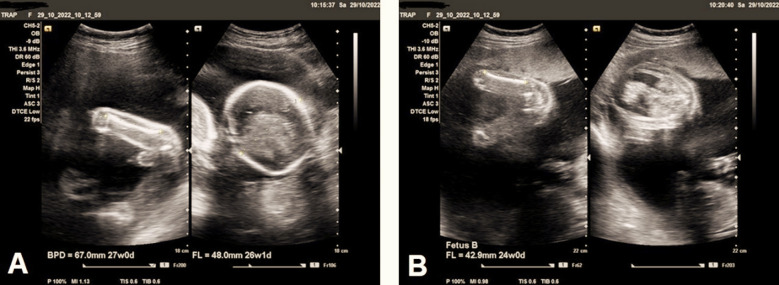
(A) Ultrasound imaging study shows the gestational age of the pump twin as 27 weeks by biparietal diameter (BPD), and 26 weeks+1 day by femur length (FL), and 27 weeks by abdominal circumference (AC), with about 983 gram expected fetal weight. The pump fetus appears structurally normal with no gross congenital anomalies with no signs of hydrops. (B) Ultrasound imaging study of the acardiac twin which was stuck to the anterior uterine superiorly by amniotic band and shows markedly under-developed upper body organs including upper limbs, upper chest, non-developed head, and non-developed heart, with around 24 weeks gestational age by femur length (FL).
